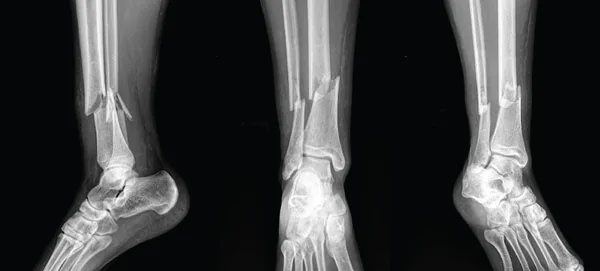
Right ankle radiograph (AP, lateral, and internal rotation views) showing a short oblique fracture of the distal third of the tibial shaft with valgus and procurvatum angulation, associated with a comminuted distal fibular fracture.
